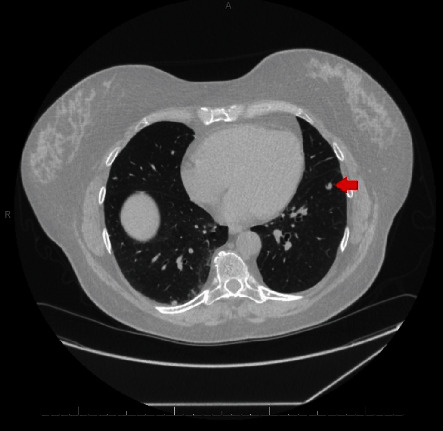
Follow-up chest CT scan showing an 8 mm left lower lobe pulmonary nodule (red arrow) which appeared to be enlarging.
